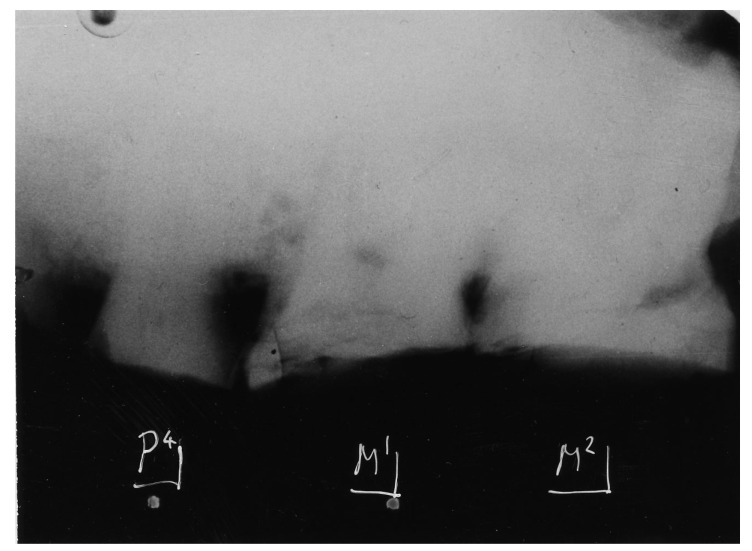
Australopithecus africanus Makapansgat MLD 9. Radiograph of maxillary teeth in situ exhibiting extreme abrasive wear of P4, M1 and M2, attesting to a tough abrasive diet early in life.
